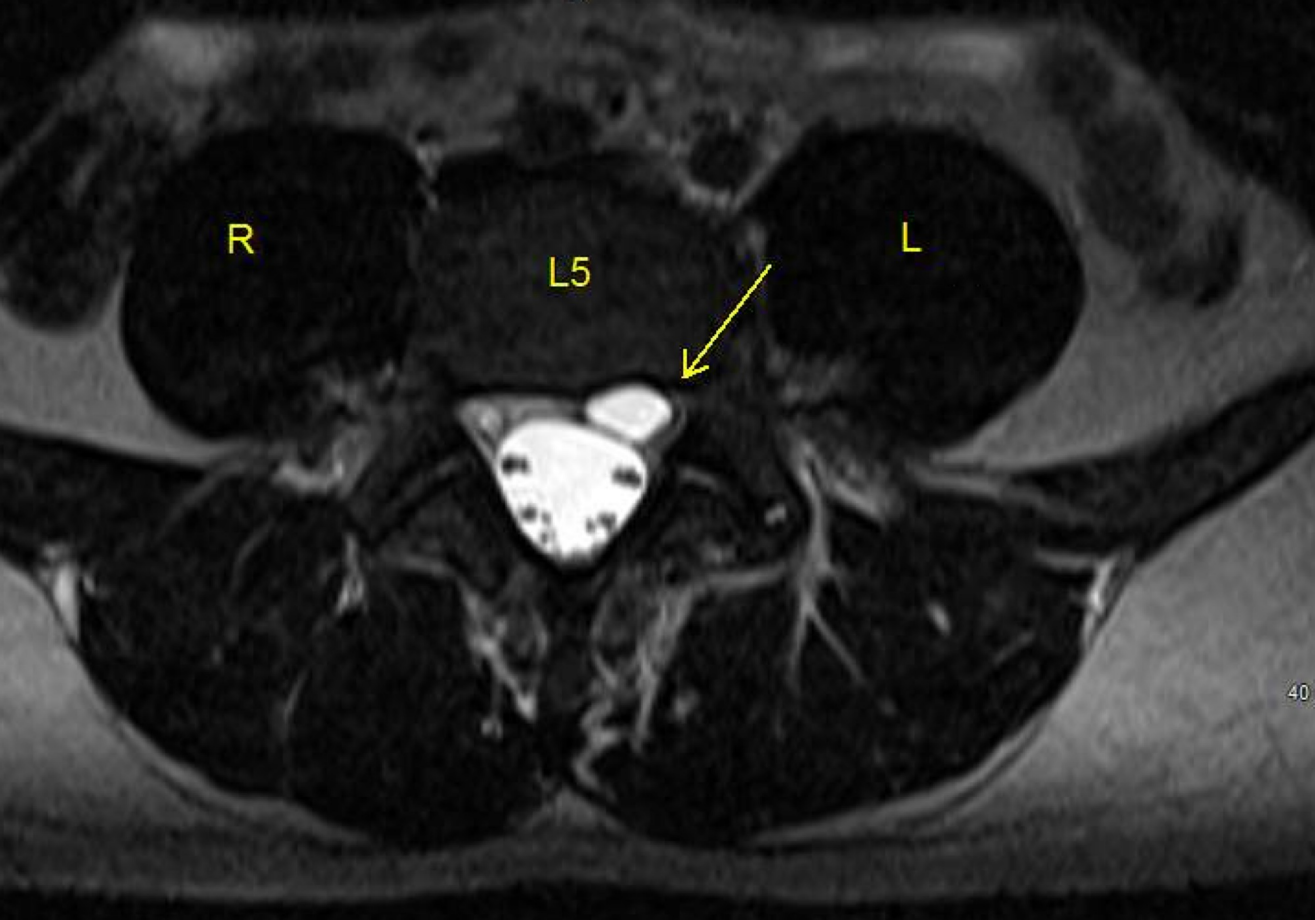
MRI T2 sequence of lumbar spine (axial view). Shows a left-sided hyperintense cystic lesion behind the L4 vertebral body (arrow).
